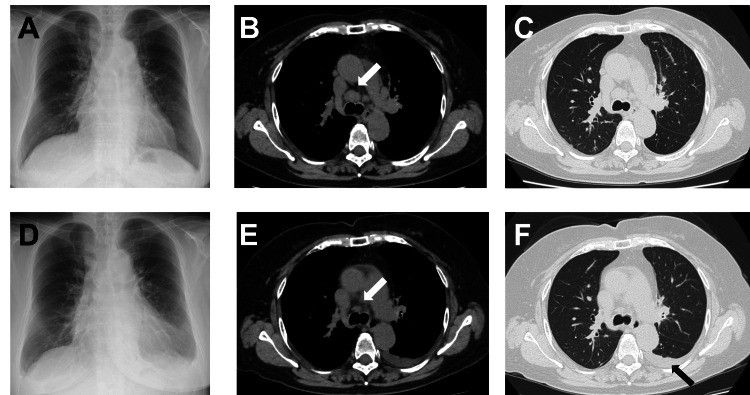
Chest X-ray and computed tomography (CT) findings before entrectinib therapy (A, B, and C) and after entrectinib therapy (D, E, and F) A: Chest X-ray showed an enlarged mediastinal shadow; B and C: Chest CT revealed multiple enlarged mediastinal lymph nodes; D: Chest X-ray showed a left pleural effusion after entrectinib treatment; E and F: Chest CT showed the reduction of multiple enlarged mediastinal lymph nodes and a new onset of minimal left-sided pleural effusion that was not present in the previous CT (arrows)
